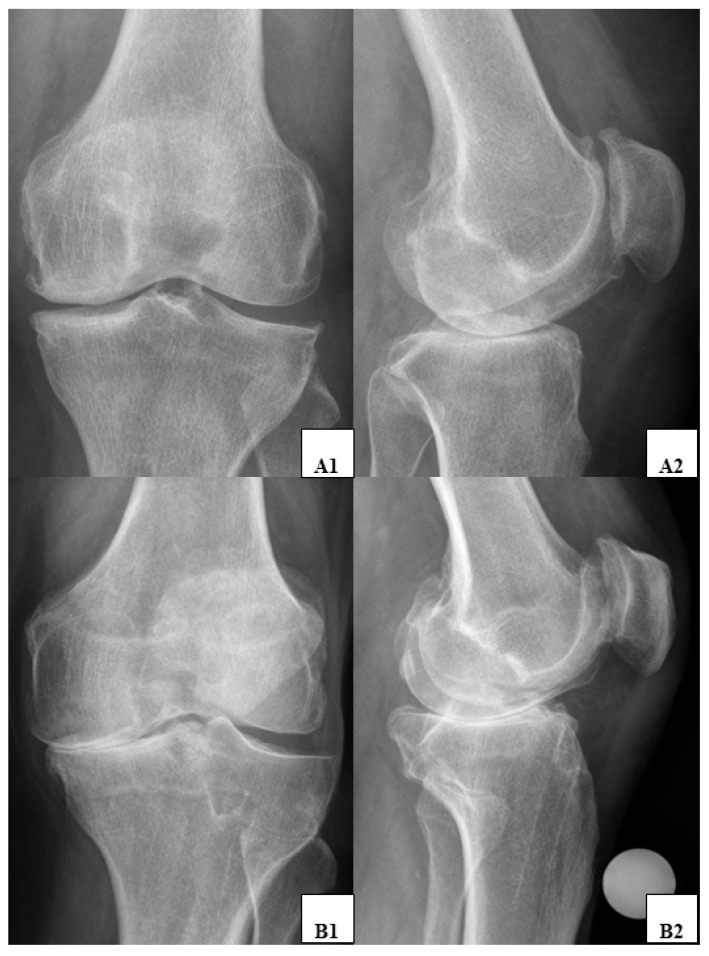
Exemplary a.p. and lateral knee radiographs from two different patients are shown. Patient A (A1,A2) exhibited poor clinical function (OKS = 15, ROM 0–10–95°) despite only moderate OA according to radiographic criteria (K&L grade 3). Patient B (B1,B2), on the other hand, showed good clinical function (OKS = 36, ROM 0–0–130°) despite severe OA according to radiographic criteria (K&L grade 4).
